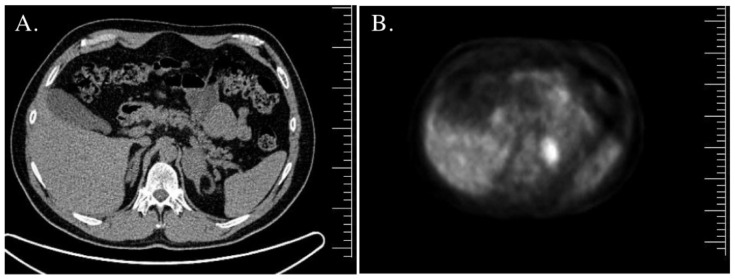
(A) Case 7—adrenal unenhanced computed tomography (CT) scan. The left adrenal tumor was 44 mm in size. (B) Case 7—Positron emitting tomography investigation using 18F-fluoro-2-deoxy-D-glucose combined with CT (^18^FDG PET/CT) with a visualized increased uptake within the left adrenal tumor.
